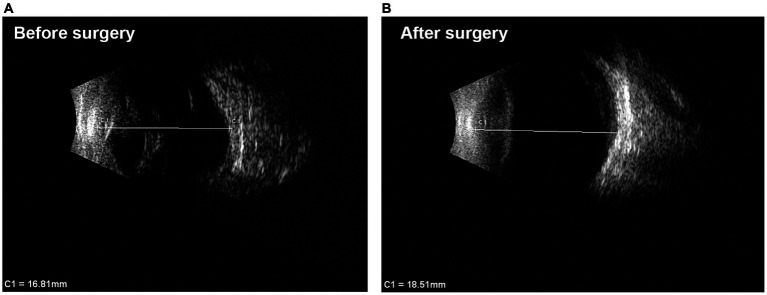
B-scan ultrasound images of one case before and after surgery. (A) Before surgery, the detached retina was a closed-closed configuration funnel. The axial length of the eye was 16.81 mm. (B) 14 months after surgery, the morphology of the eye became better and the axial length was 18.51 mm. The detached retina was open well although its thickness and stiffness make it difficult to become completely flat. Above all, the eye was saved and phthisis bulbi did not happen after the surgery.
